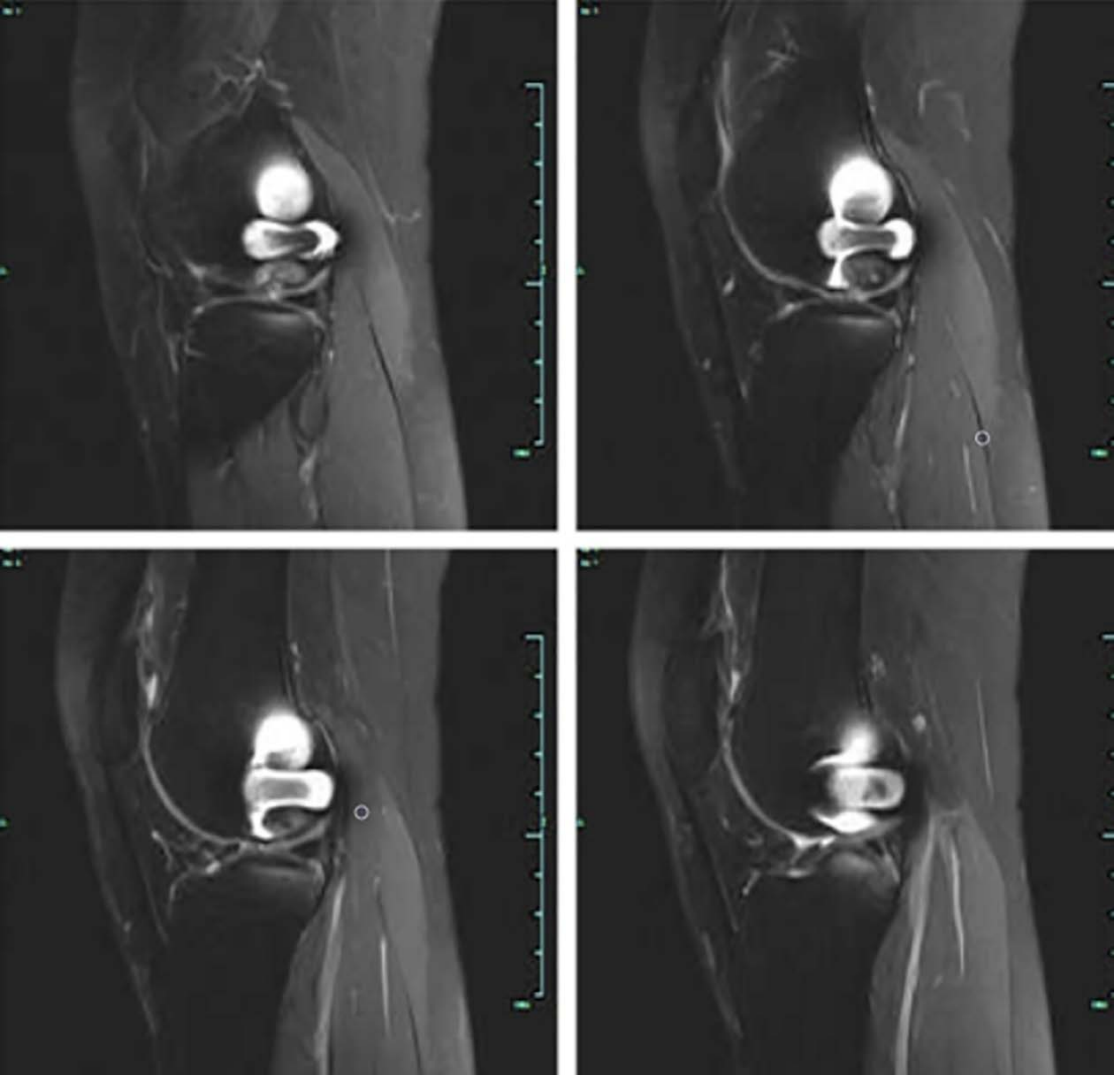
Sagittal MRI images were reexamined 18 months after operation, MRI = magnetic resonance.
